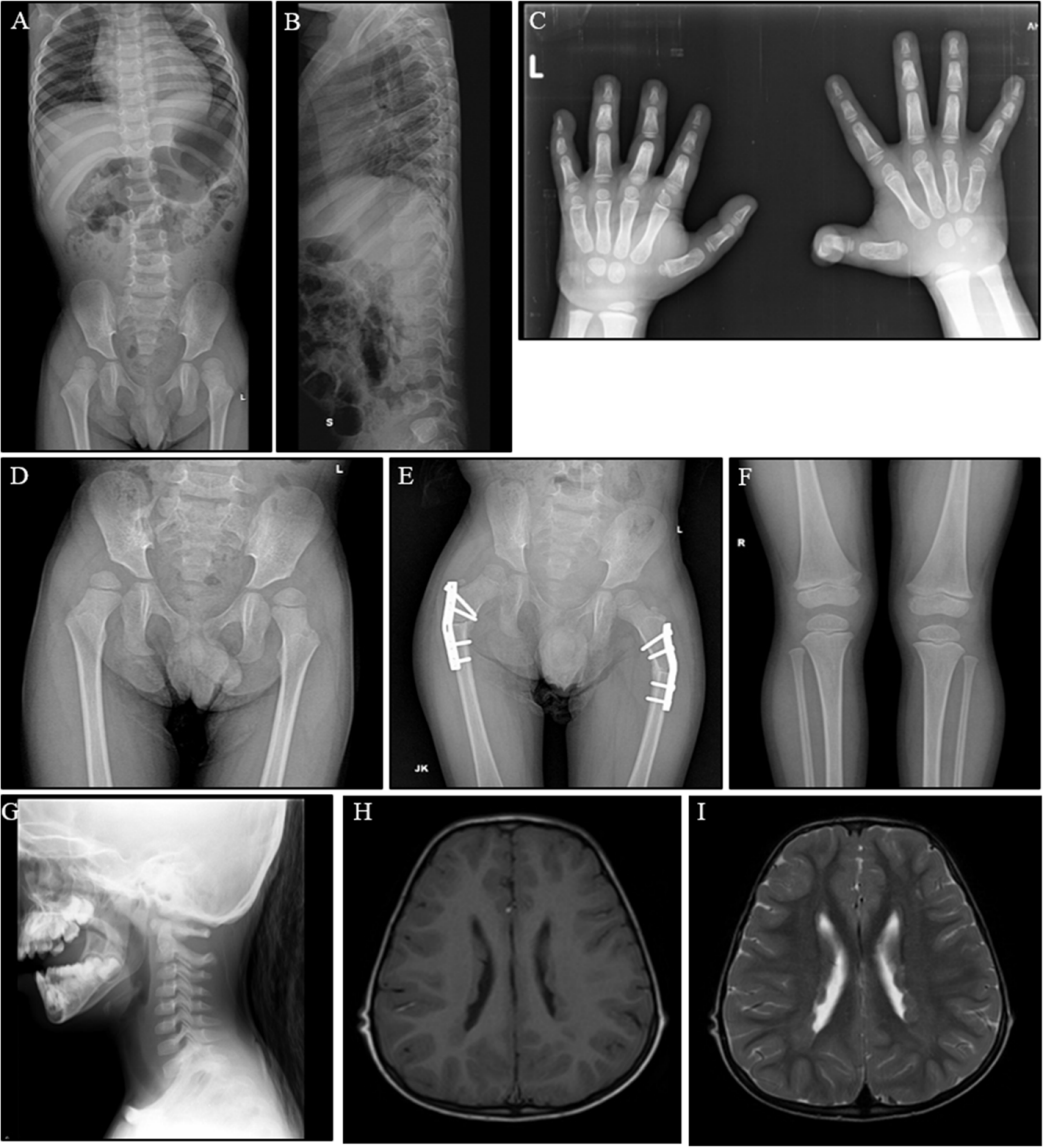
Skeletal survey of five-year old Indian patient (case A). a, b, g At 5 years of age the skeletal survey revealed tall vertebral bodies, c delayed ossification of carpal bones, dislocated terminal phalanx of right thumb, d, e bilateral hip subluxation for which he underwent bilateral varus derotation osteotomy, f genu recurvatum and (h, i) magnetic resonance imaging of the brain showed PVNH along the subependymal regions of both lateral ventricles
